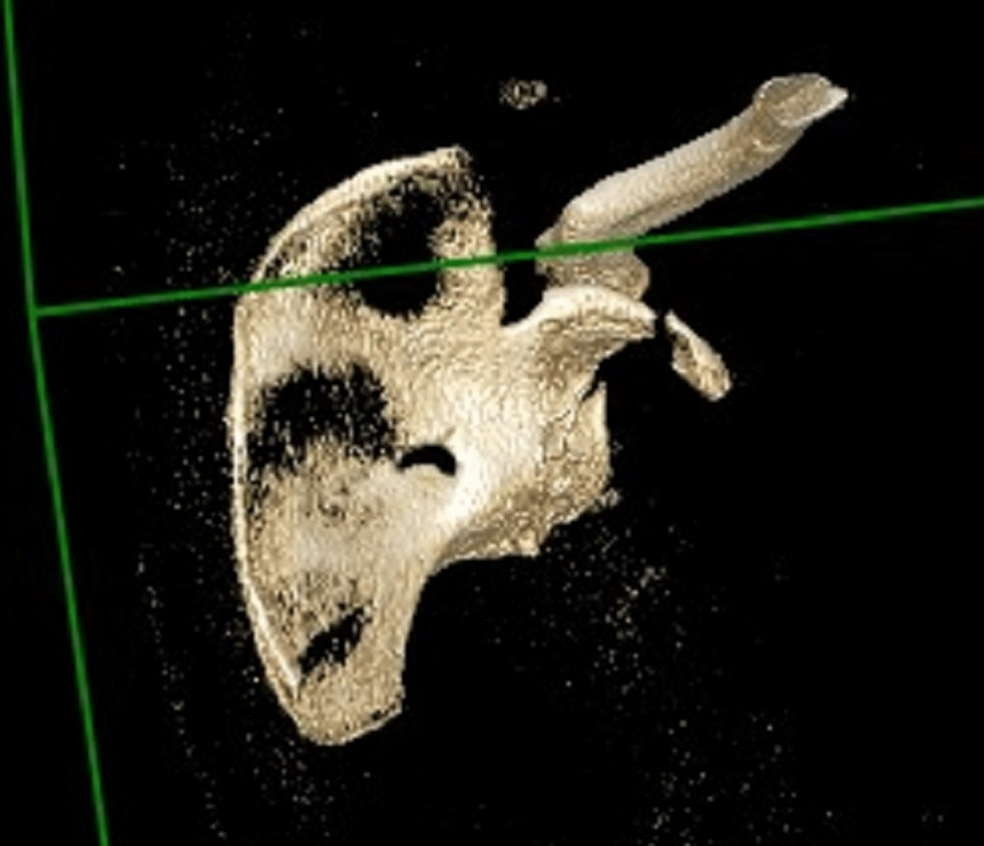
CT reconstruction of the coracoid process fracture Viewed from the anterior, this image demonstrates the large coracoid process fracture and the relevant displacement.
